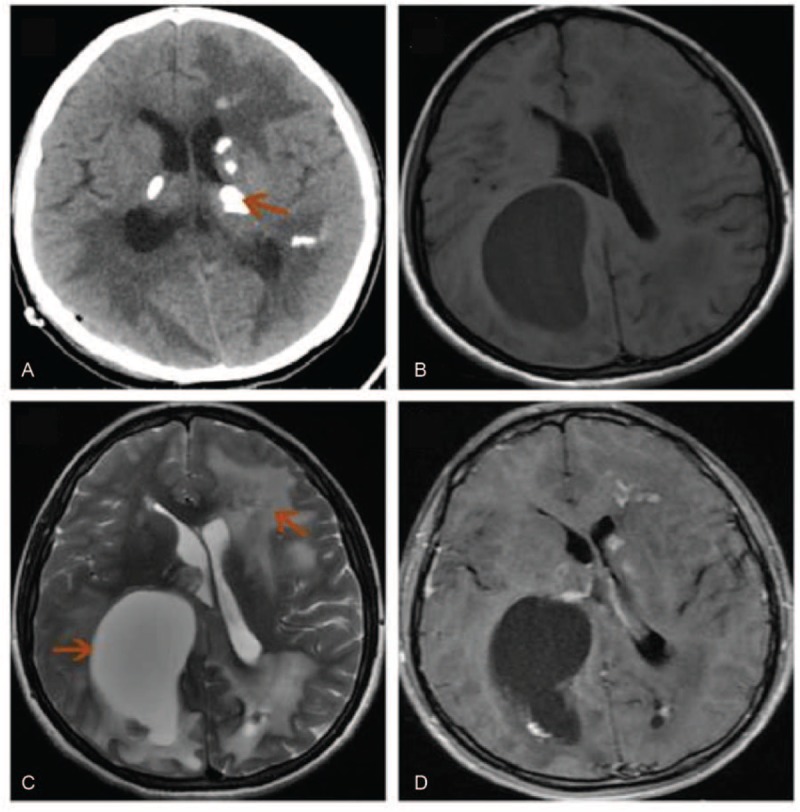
Radiological profiles of Case 1. (A) Brain computed tomography showed multiple asymmetric calcifications in the bilateral basal ganglia. (B) Magnetic resonance T1-weighted imaging showed a giant cyst in the right parietal lobe and hypointensities in the bilateral white matter. (C) Magnetic resonance T2-weighted imaging showed a giant cyst in the right parietal-occipital lobe and extensive hyperintensities in the bilateral white matter. (D) Magnetic resonance contrasted T1-weighted imaging showed nodular enhancement along the cyst wall and focal enhancement in the white matter.
